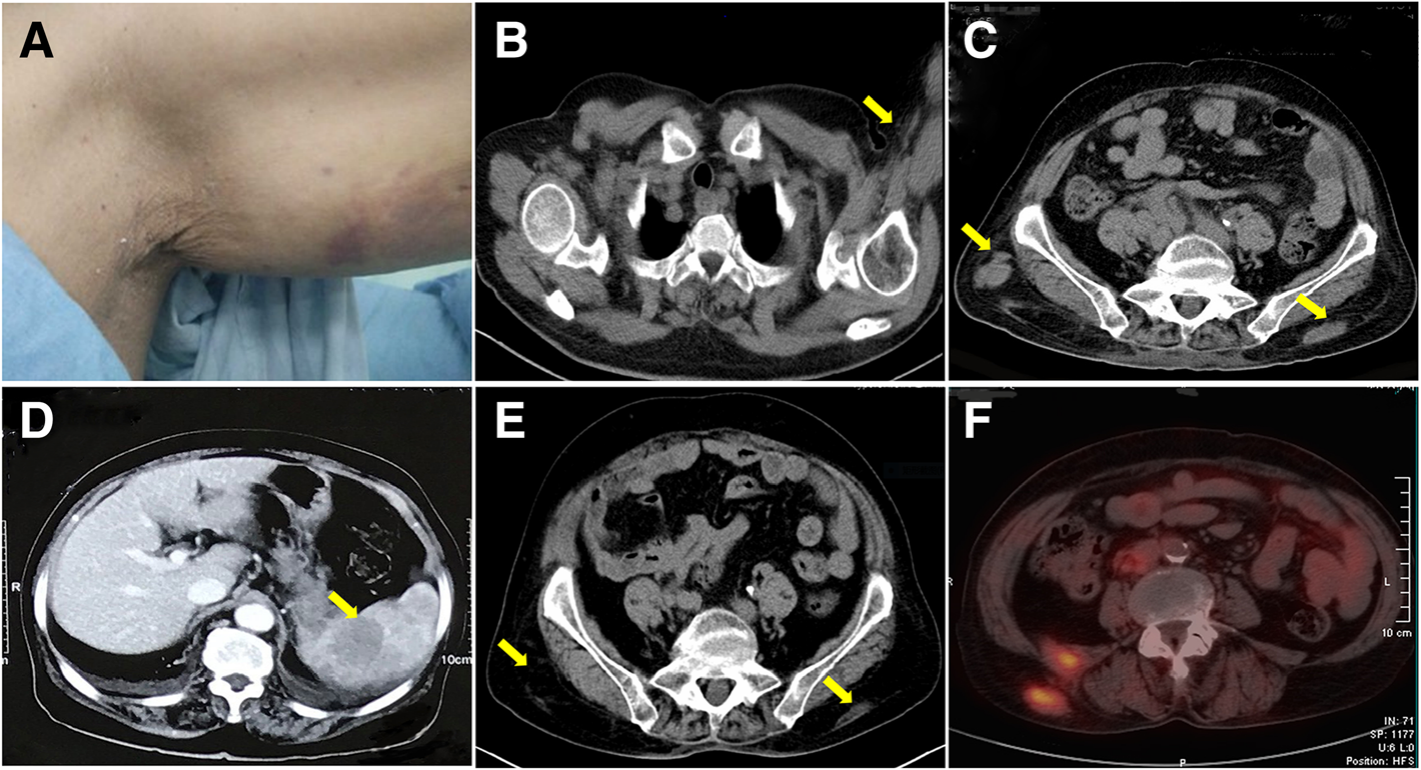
General observation and imaging examinations. (a): Subcutaneous mass at the left upper arm. (b-d): CT scans before the treatment. (b): A 2.0 cmx1.6 cm subcutaneous poor-defined tumour (arrow) at the left upper arm. (c): Several ill-defined soft tissue density lesions (arrows) at the waist. (d): Multiple low-density plaques (arrow) in the enlarged spleen by abdominopelvic contrast-enhanced CT. (e&f): Smaller lumbar masses (arrows) by PET-CT at the nine-month follow-up
